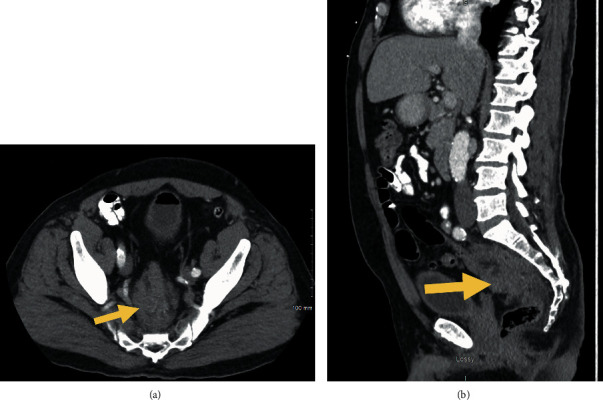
(a) Axial abdomen/pelvis CT showing a soft tissue mass (arrow) inseparable from the rectal wall. (b) Sagittal abdomen/pelvis CT showing a soft tissue mass (arrow) inseparable from the rectal wall.
